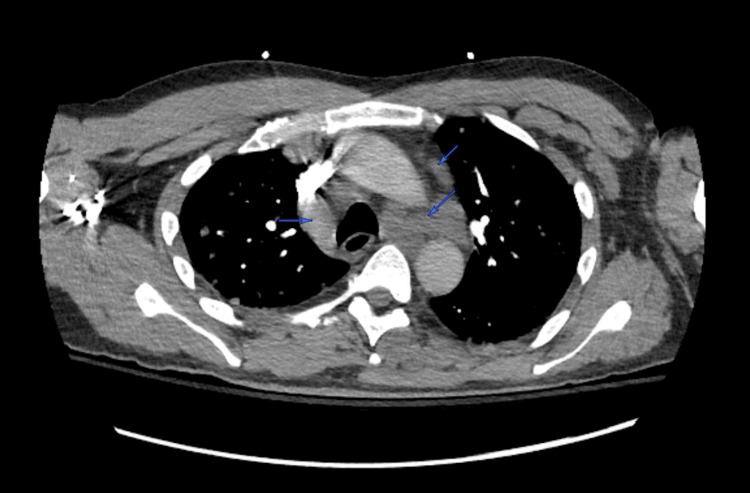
Axial CTA scan of the chest revealing mediastinal, left hilar, and aortocaval lymphadenopathy (blue arrows) CTA: computed tomography angiography
